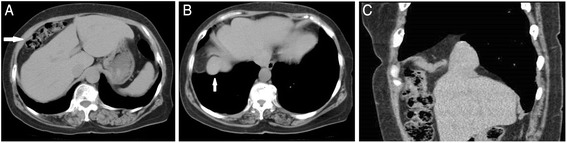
a Computed tomography (CT) of the abdomen showing the transverse colon located above the right lobe of the liver (*arrow*). b CT showing a small part of the liver herniated into the right thoracic cavity (*arrow*). c Sagittal view of the same CT showing a small part of the liver and intestinal fat tissue in the right thoracic cavity
